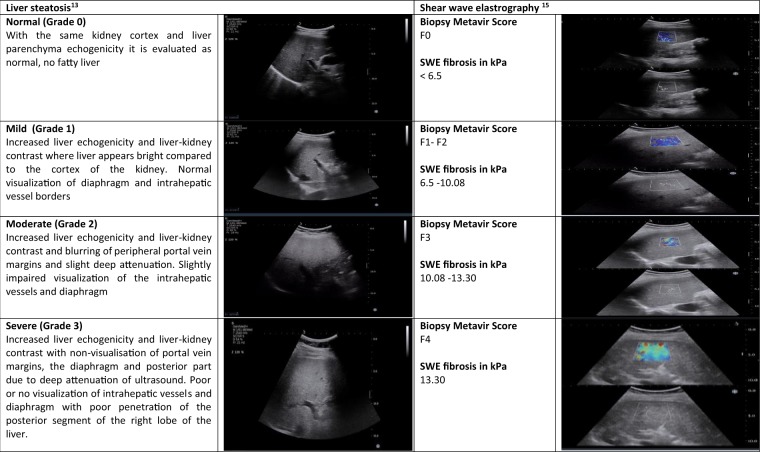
Ultrasonography assessment of steatosis and shear wave elastography of liver.
